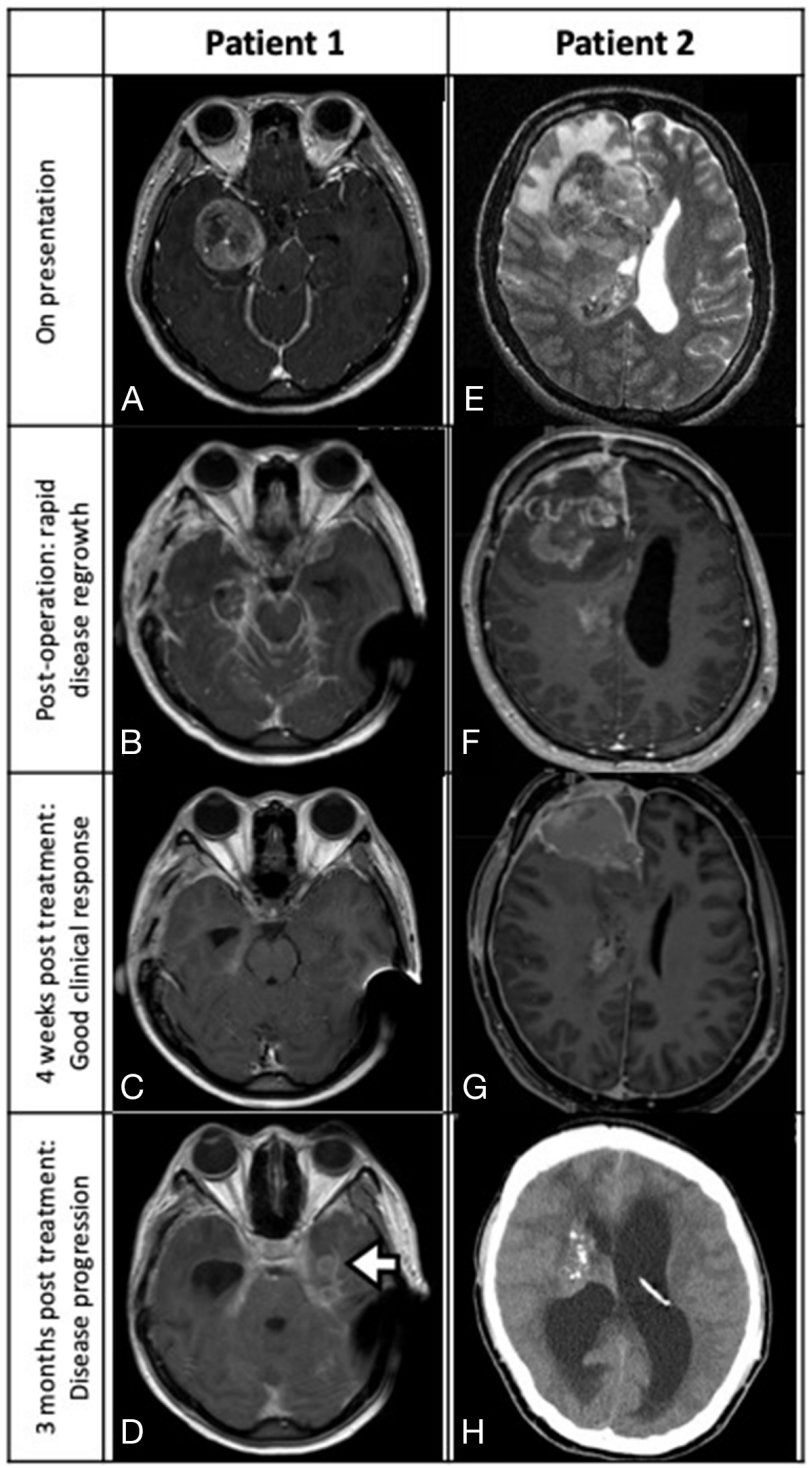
Patient 1 (A-D): MRI depicting a right temporal glioblastoma with ambient cistern LMS (A, axial T1-weighted contrast-enhanced sequence). Post-near total resection three-week MRI showing local recurrence with diffuse LMS (B, axial). After receiving four weeks of dabrafenib and trametinib, significant tumor regression was noted (C, axial MRI). Three months after starting combined target therapy, LMS with a new left temporal lesion was detected (D, axial MRI; white arrow, multifocal tumor recurrence). Patient 2 (E-H): MRI scan revealing a right frontal glioblastoma with spread into the body of the right lateral ventricle (E, axial T2-weighted sequence; white arrowhead, ventricular tumor). Post-subtotal resection MRI showing rapid regrowth of tumor at surgical cavity and the development of communicating hydrocephalus (F, sagittal T1-weighted contrast-enhanced sequence). Significant tumor regression observed four weeks after starting vemurafenib (G, axial T1 contrast enhanced MRI). Disease rapidly progressed after stopping BRAF inhibitor and developed severe hydrocephalus (H, plain CT).
